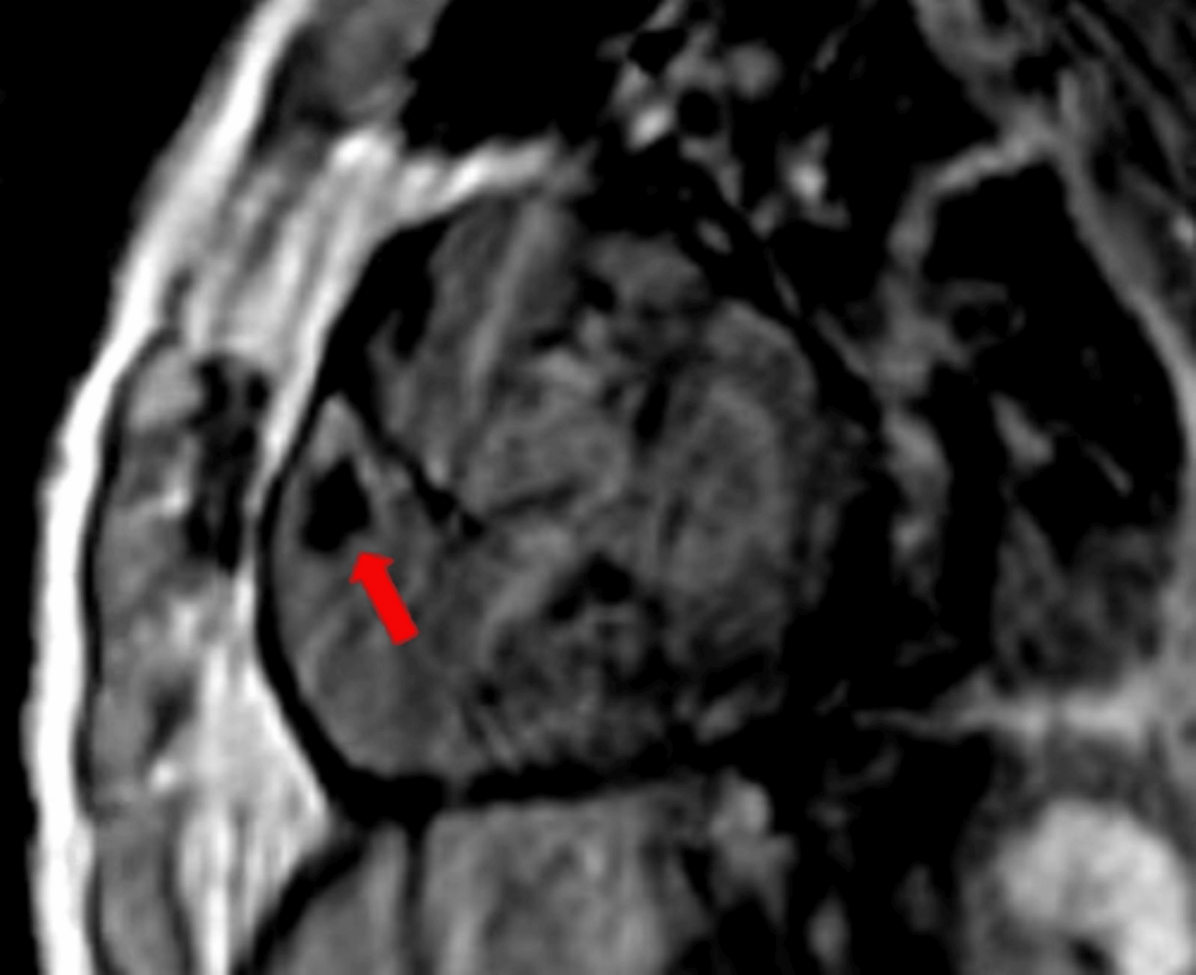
Cardiac magnetic resonance imaging in the short-axis view shows a 2.1 cm x 1.5 cm mass with no enhancement on a Ti600 sequence consistent with a thrombus
